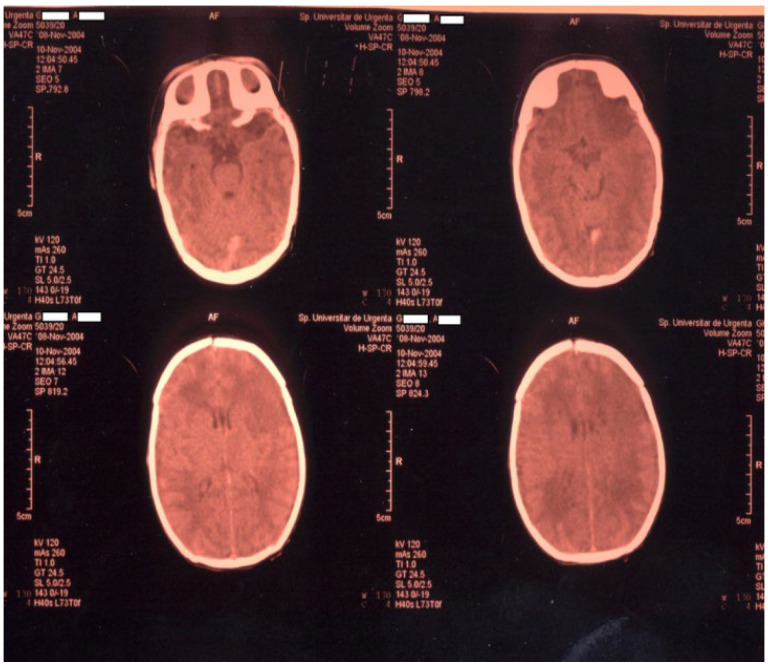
CT examination for newborn #1.
